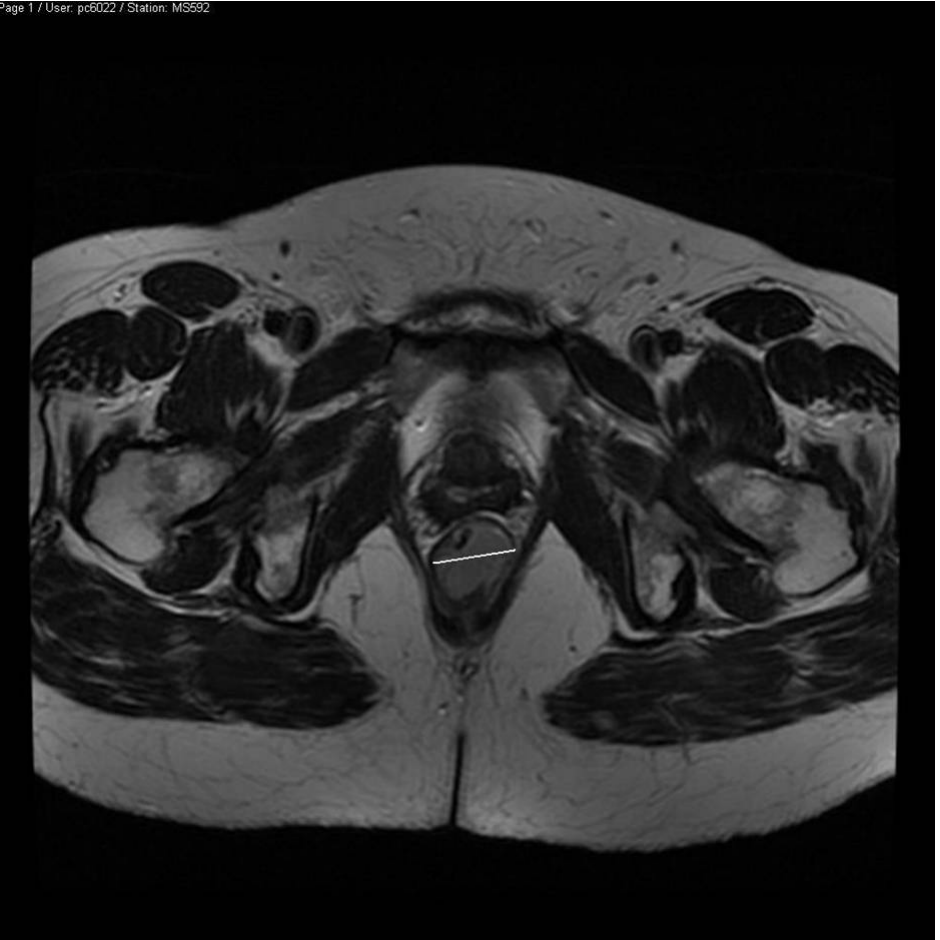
MRI of the anal canal showing the tumour.
